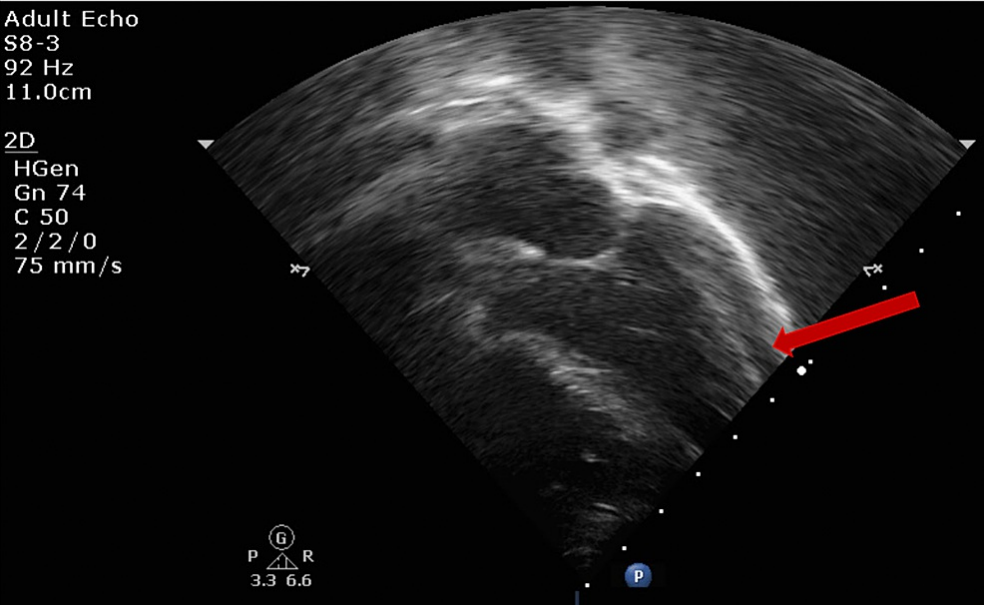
Transthoracic echocardiogram (TTE) subxiphoid short-axis view showing trivial pericardial effusion
